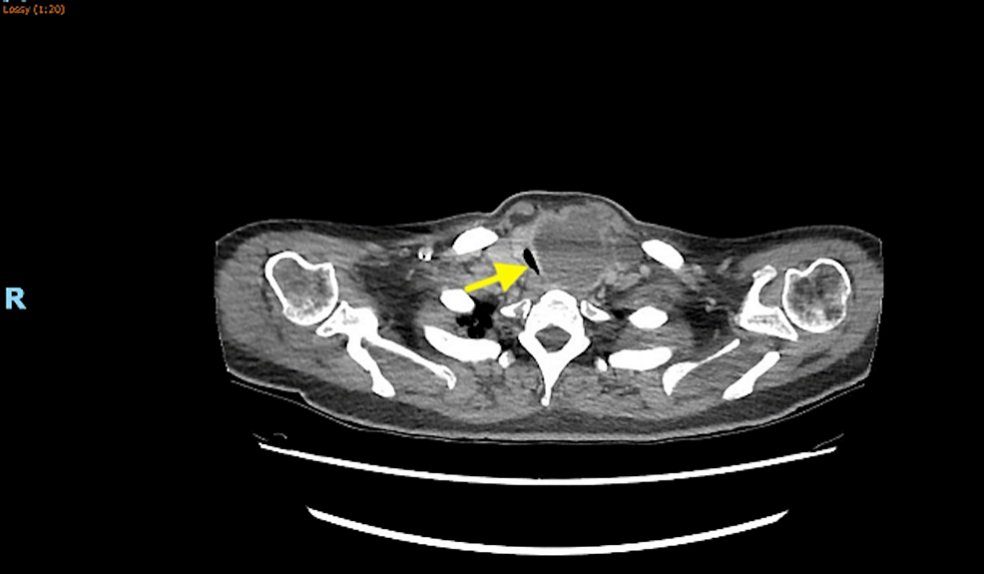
Axial view of the thoracic computed tomography scan showing significant tracheal compression and narrowing.
